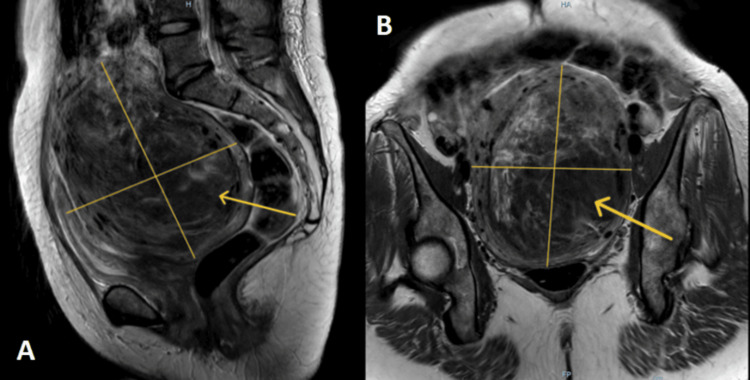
Magnetic resonance imaging (MRI) of the abdomen pelvis shows a large 13.1 cm intramural uterine fibroid Grade 4 (yellow arrows).
